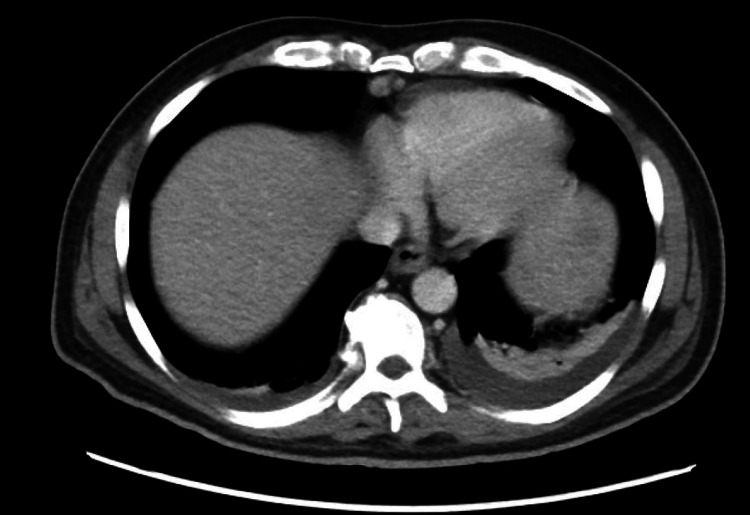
CT scan of abdomen and pelvis demonstrating small bilateral pleural effusions, more pronounced on the left side
